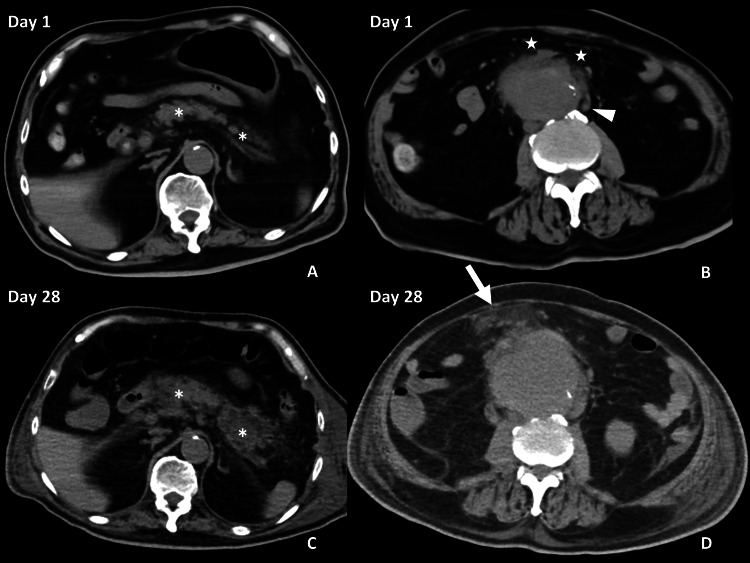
Plain abdominal CT on day one (A and B), and on day 28 (C and D) CT showed an abdominal aortic aneurysm (AAA) with an unclear margin (B, star) and paraspinal lymph node swelling (B, arrowhead) on arrival. The pancreas showed a normal appearance (A, asterisk). On day 28, the AAA expanded with the dirty fat sign observed on the ventral side (D, arrow). The pancreas became swollen (C, asterisk).
